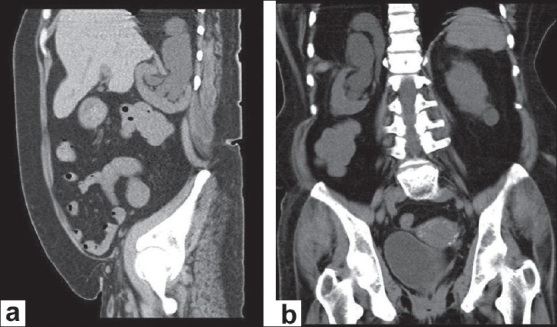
Noncontrast-enhanced computed tomography scan; (a) Saggital images showing malrotated and cranially displaced right kidney with ureter and retroperitoneal fat herniating into thorax; (b) Coronal images showing right ureter looping into thorax and back into retroperitoneum through a diaphragmatic defect
